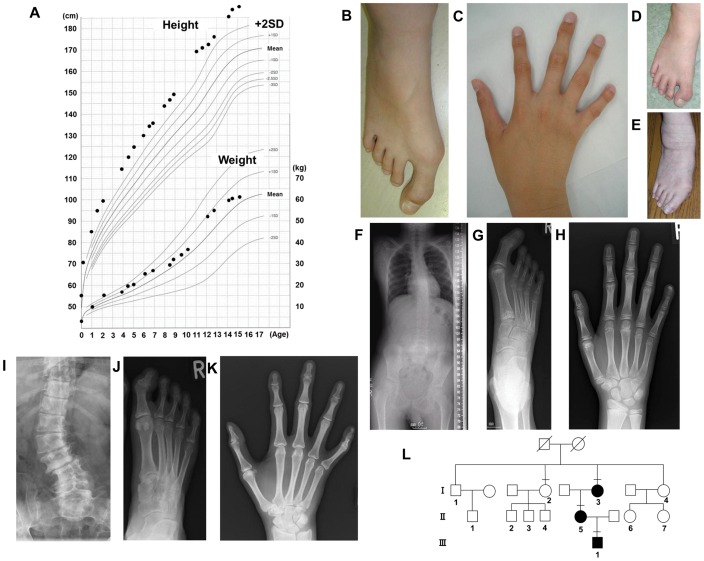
Clinical manifestations of the family. (A) Growth curve of the proband. The X- and Y- axis indicate age (year) and height (cm) or weight (kg), respectively. The bold line represents the mean for Japanese males. (B, C) Photographs of the proband’s right foot (B) and hand (C). The great toe was markedly long and wide. The fifth distal phalanx of the hand also showed minor clinodactyly. He has given written informed consent to publication of his photographs. (D, E) The right foot of the mother (D) and grandmother (E), respectively. The mother’s great toes were surgically shortened at 15 years of age. The grandmother’s great toes were large in early life and shortened due to arthrogryposis with aging. They have given written informed consent to publication of their photographs. (F–H) Radiographs of the proband’s skeleton. His spine showed mild scoliosis (F). The great toe was markedly long and wide (G), and the fifth distal phalanx of the hand showed minor clinodactyly (H). (I–K) Radiographs of the mother. Severe scoliosis and lumbar vertebra fractures (I) as well as a markedly long and wide great toe (J), and minor clinodactyly in the fifth digit of the hand (K) were observed, like in the proband. (L) Family tree. III-1, II-5, and I-3 indicate the proband, mother, and maternal grandmother, respectively, who had the phenotype. I-2 indicates a maternal grand-aunt, who had no phenotype and was included in the present study.
